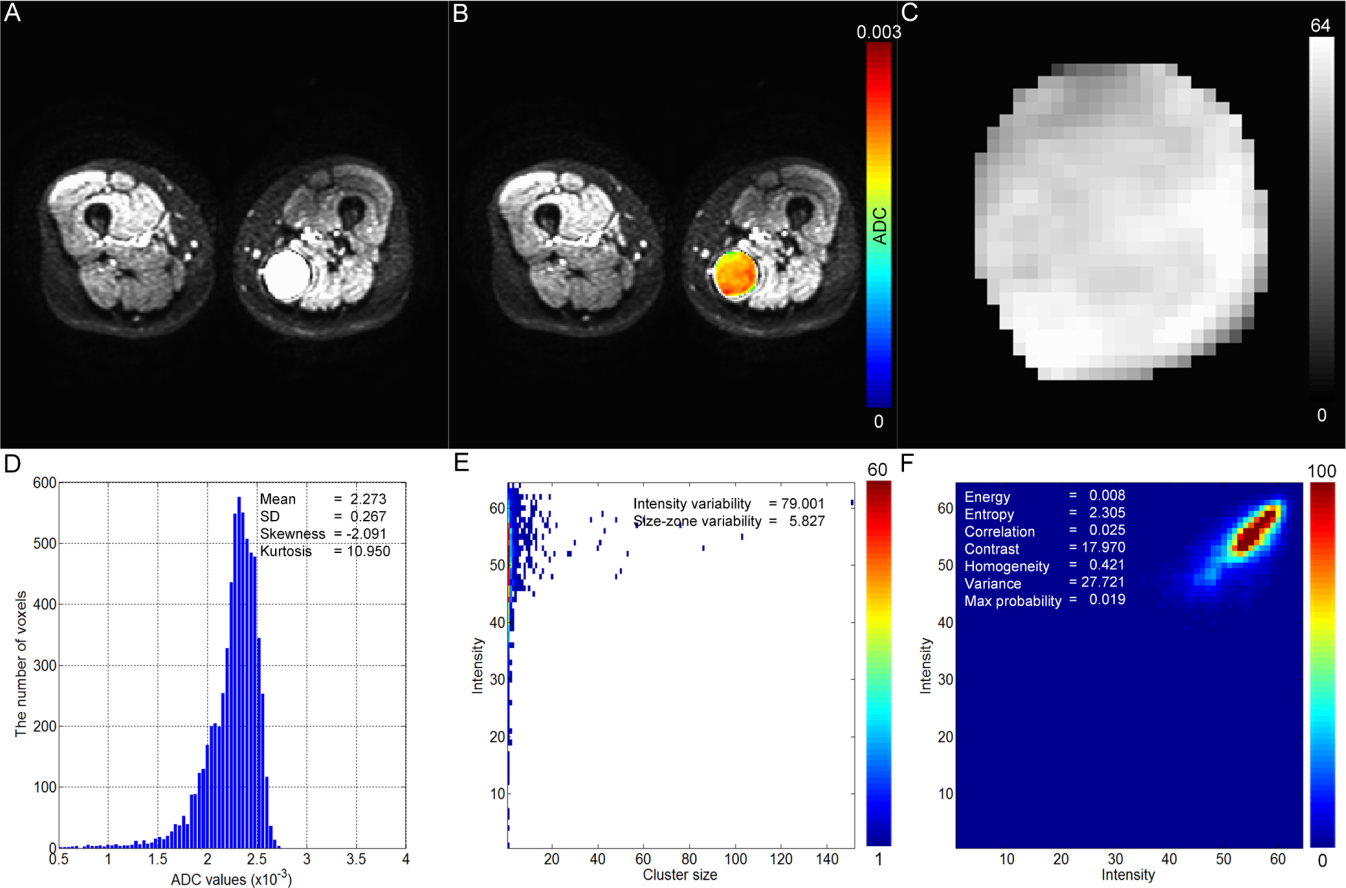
A case of malignant myxoid-containing STT (myxoid liposarcoma) in a 52-year-old female. DWI (A) shows a circumscribed high signal intensity mass in the left posteromedial thigh. Using a color ADC map (B), a discrete ADC map with 64 different values ranging from 1 to 64 (C), a histogram (D), a GLSZM (E), and a GLCM (F) were generated.
